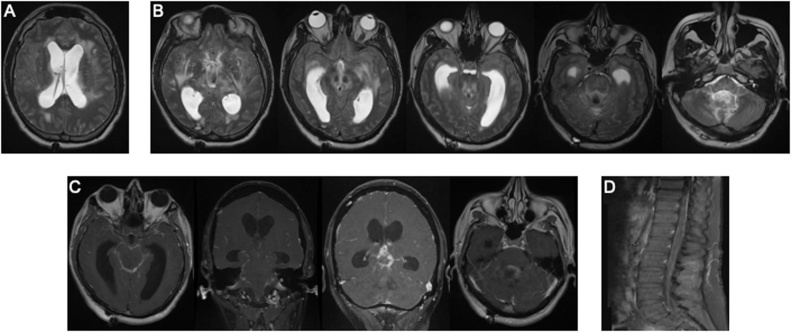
Magnetic resonance imaging of the neuraxis. A. Axial T2-weighted image showing hydrocephalus and transependymal flow. B. Representative T2-weighted axial images showing hyperintensity in bilateral insula, frontal lobes, posterior limbs of the internal capsules and thalami, extending inferiorly into the midbrain, pons, cerebellar peduncles, and medial cerebellar hemispheres. C. T1-weighted, contrast-enhanced images showing diffuse leptomeningeal enhancement and thickening within the basilar cisterns and upper cervical canal, pineal region and fourth ventricle. D. T1-weighted, contrast-enhanced sagittal scan of the thoracolumbar region showing diffuse leptomeningeal enhancement in the thecal sac.
